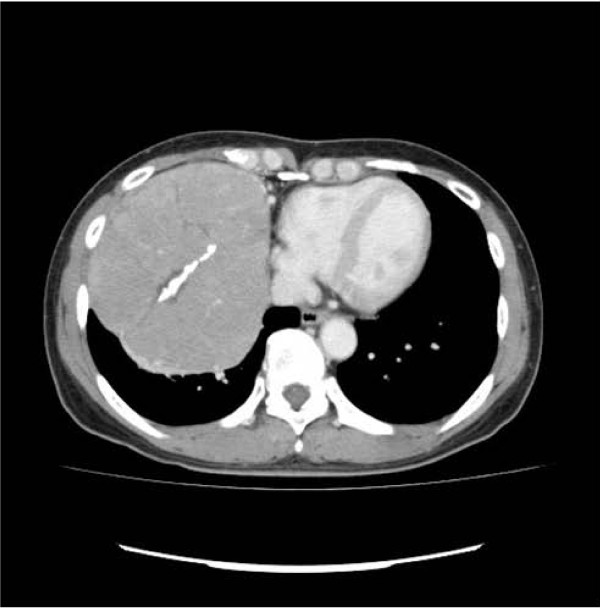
Chest computed tomograph showing a solid tumor (15 × 10 × 8 cm) with a clear borders and internal calcification in the right thoracic cavity.
